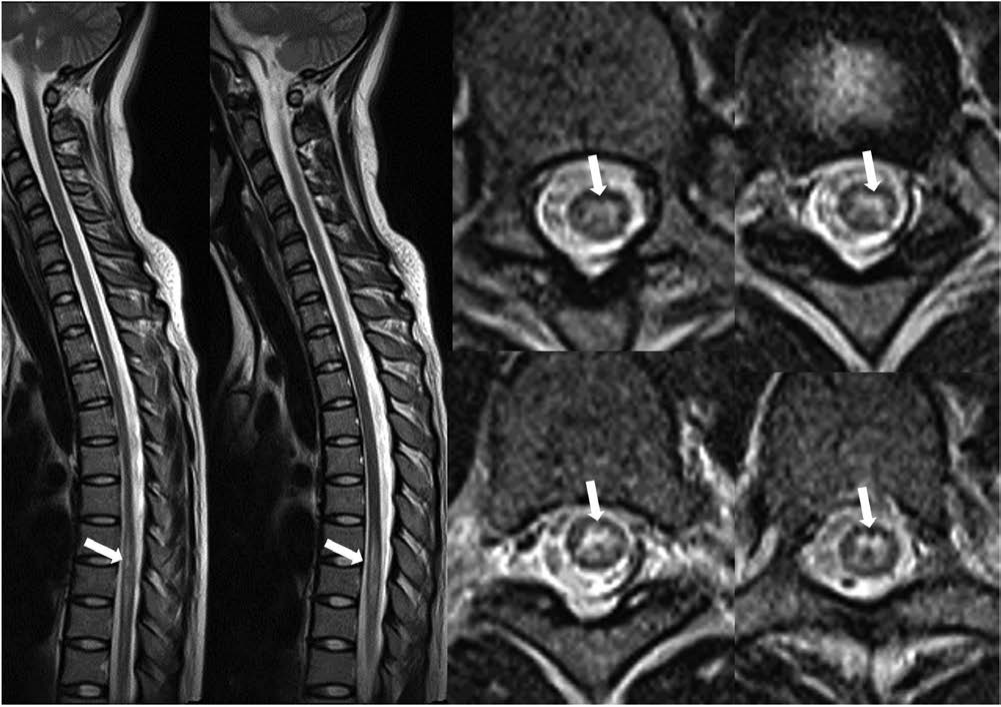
Spinal cord magnetic resonance imaging (MRI) in the 2^nd^ wk of hospitalization. Longitudinal extended transverse myelitis (LETM) lesion of T2 hyperintensity in thoracic spinal cord T7–8-9 level.
